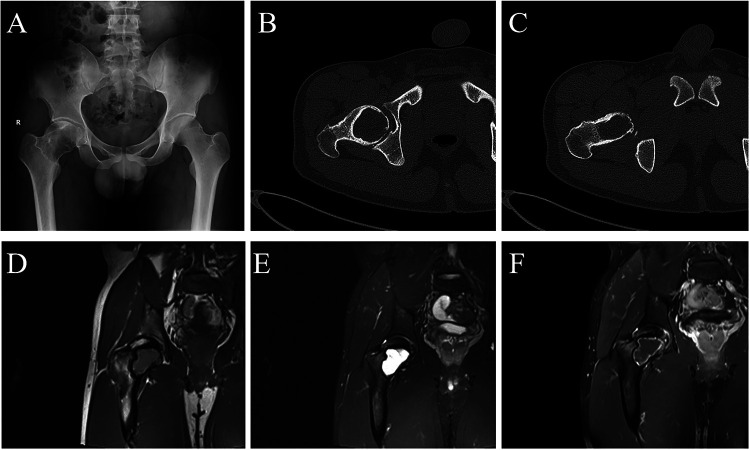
Preoperative imaging findings of the proximal femur. (A) DR showing a low-density lesion in the proximal femur. (B,C) CT demonstrating cystic low-density lesions involving the femoral head and neck, with marginal sclerosis. (D–F) MRI revealing an isointense signal on T1-weighted images and a hyperintense signal on T2-weighted images.
